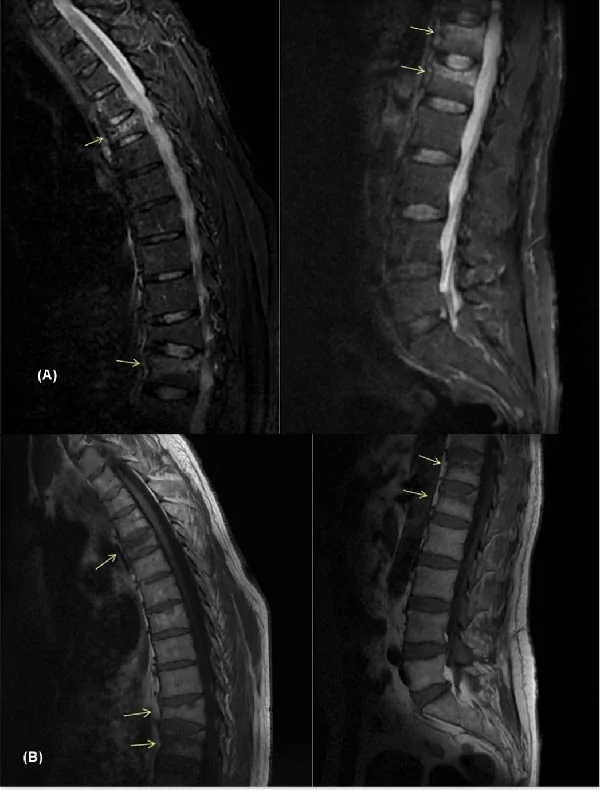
Sagittal MRI of the thoracolumbar spine showing multiple vertebral compression fractures. (A) Short tau inversion recovery (STIR) sequence demonstrating acute fractures at T5, T12, and L1 (yellow arrows) with marrow edema indicating acuity, and a subacute T11 fracture showing intermediate signal. (B) Corresponding T1‐weighted images showing hypointense signal at T5, T11, T12, and L1, consistent with acute and subacute fractures.
